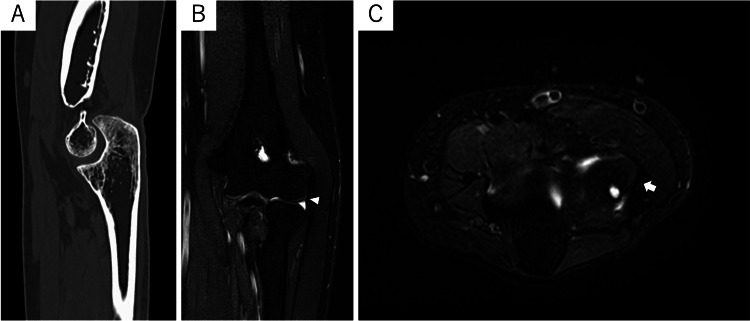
Preoperative CT and MRI scans of the left elbow of case one (A) No osteoarthritic changes were found in the CT scans. (B) The coronal T2-weighted fat suppression MRI showed a normal signal intensity of the LCL (white arrowhead). (C) The axial T2-weighted fat suppression MRI showed a normal signal intensity of the proximal attachment of the lateral collateral ligament (LCL) (white arrow).
